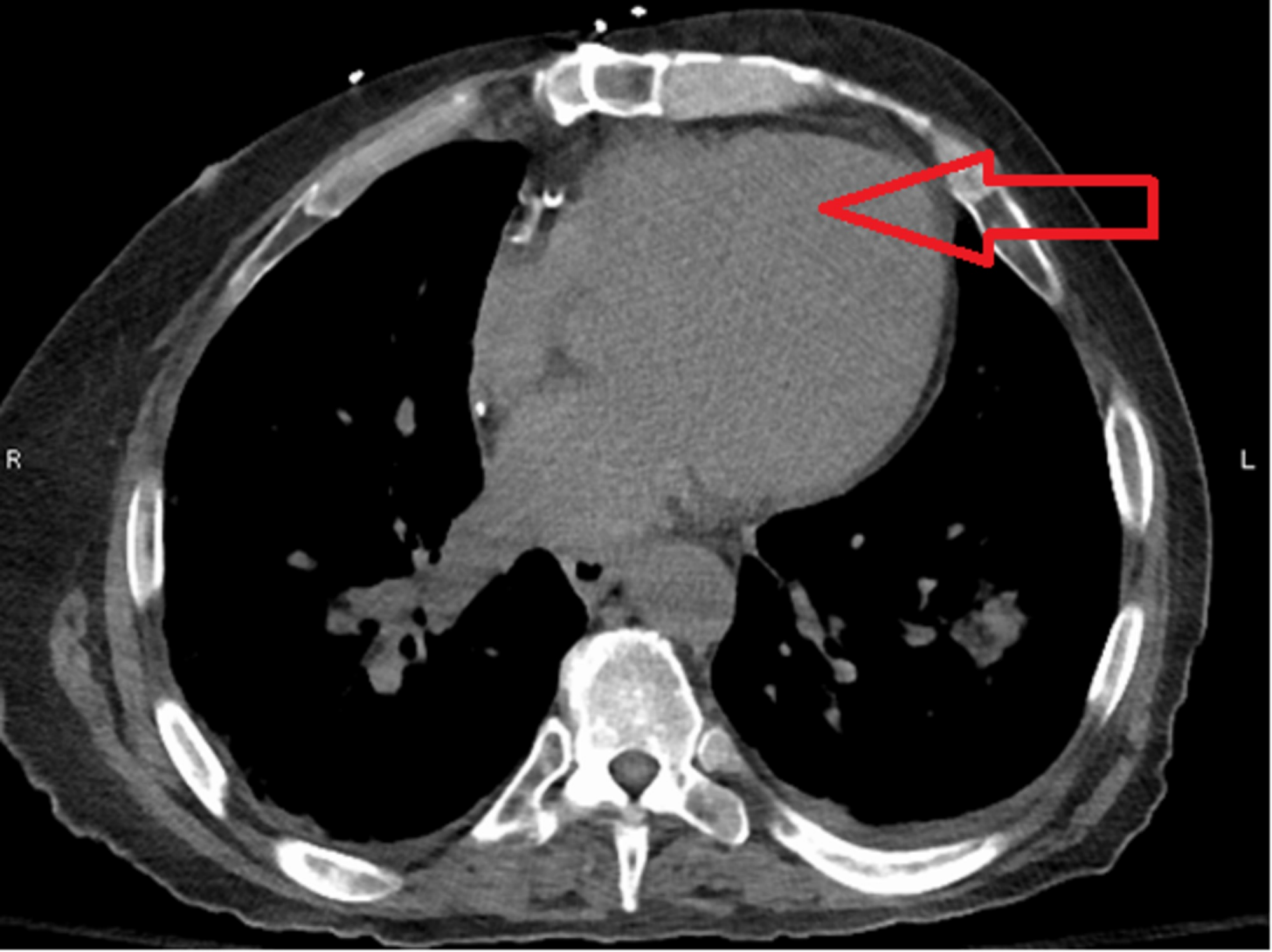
Computed tomography (CT) scan of chest showed resolution of air embolism in left ventricle
